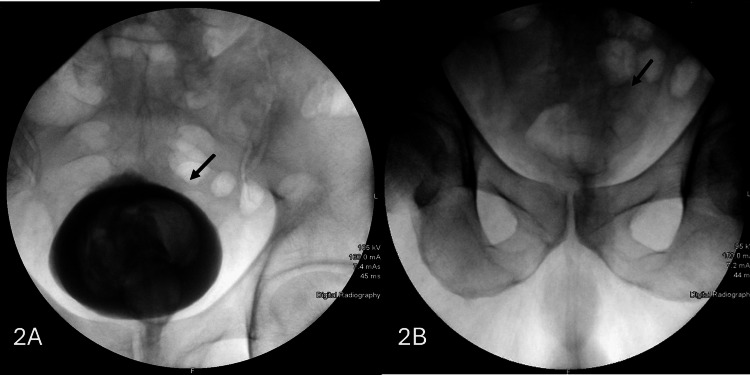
X-ray cystogram during (A) the filling phase with no extravasation and (B) during the voiding phase.
